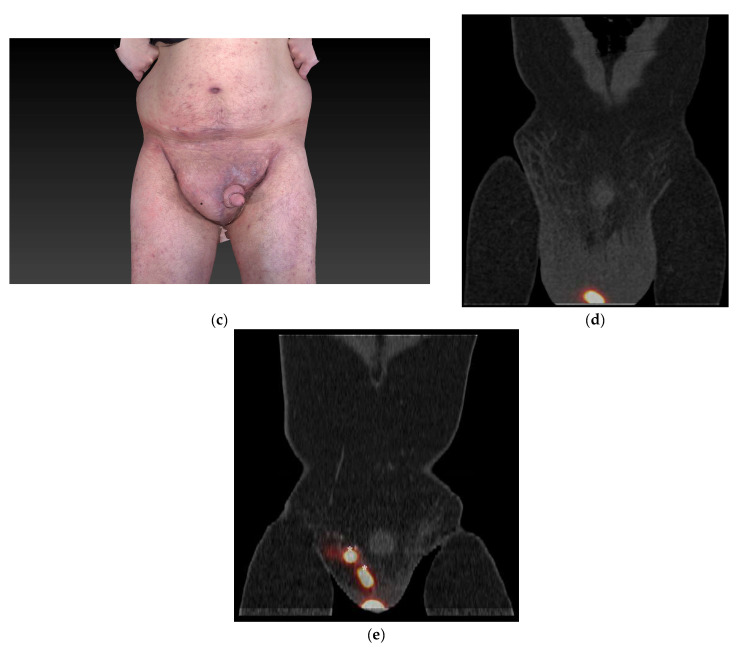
Images of advanced stage III penoscrotal lymphedema (PL), according to the classification in Table 1, (patient 8) (a) before treatment, (b) during surgery, and (c) after treatment. Panels (d,e) show low-dose SPECT-CT scans, before and after surgery. At initial presentation, the patient suffered from severe local infection and folliculitis and was therefore treated with long-term antibiotics (a). Surgery included resection and flap reconstruction of scrotum and penis combined with a vascularized lymph node transfer (VLNT) into the scrotum (b), with the superficial external pudendal artery and vein as recipient vessels (marked with an asterisk). Panel (c) shows the result 8 months after surgery. The monitoring island of the VLNT in (c) is marked with an asterisk. The skin and subcutaneous tissue of the island may be removed 6 months after surgery to further reduce the volume of the newly fabricated scrotum. Lymphoscintigraphy, including low-dose SPECT-CT scans, showed significantly improved lymphatic transport of the scrotum 3 months after surgical intervention (e), when compared to preoperative findings (d). The transplanted lymph nodes in (e) are marked with an asterisk.
